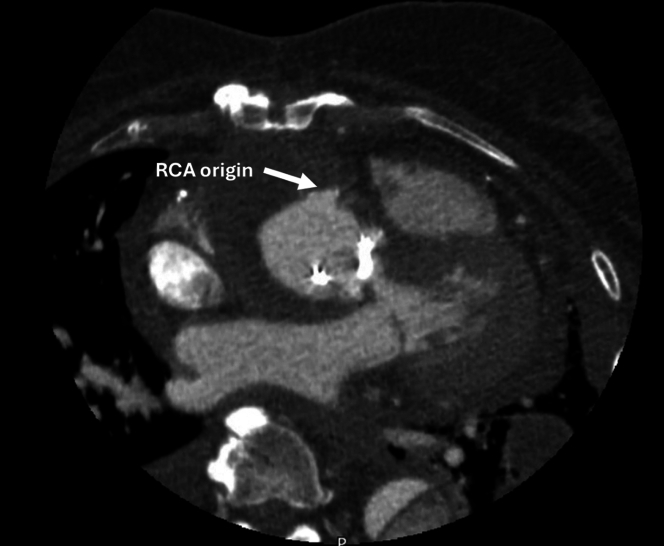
Repeat Cardiac Gated Computed Tomography 4 Months Later Demonstrating Resolution of the Pseudoaneurysm
